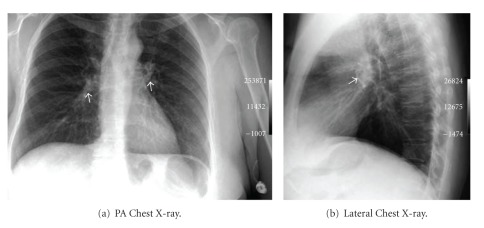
(a) The PA chest X-ray demonstrates the catheter fragment superimposed upon the mediastinum with portions of the catheter seen in both hilar regions (arrows). The arm port and the residual catheter are seen in the arm, cranial to the antecubital fossa, on the margin of the image. (b) The lateral chest X-ray demonstrates the catheter fragment superimposed upon the mediastinum (arrow).
